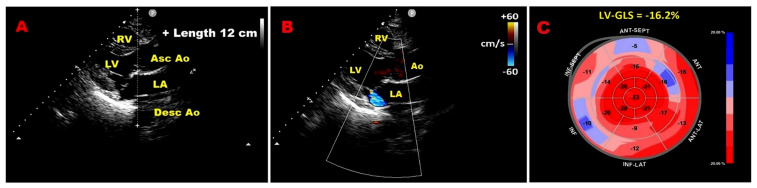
Example of MVP with concave chest wall conformation (MHI > 2.5 or A-P diameter ≤ 13.5 cm). (A) Narrow A-P thoracic diameter (≤13.5 cm) measured from the parasternal long-axis view. (B) Parasternal long-axis view showing mild MR on color Doppler. (C) 2D–STE bull’s eye plot revealing mild-to-moderate impairment of LV–GLS, most marked in basal segments. 2D, two-dimensional; Ao, aorta; A-P, antero-posterior; Asc, ascending; Desc, descending; GLS, global longitudinal strain; LA, left atrium; LV, left ventricle; MHI, Modified Haller Index; MVP, mitral valve prolapse; MR, mitral regurgitation; RV, right ventricle; STE, speckle tracking echocardiography.
